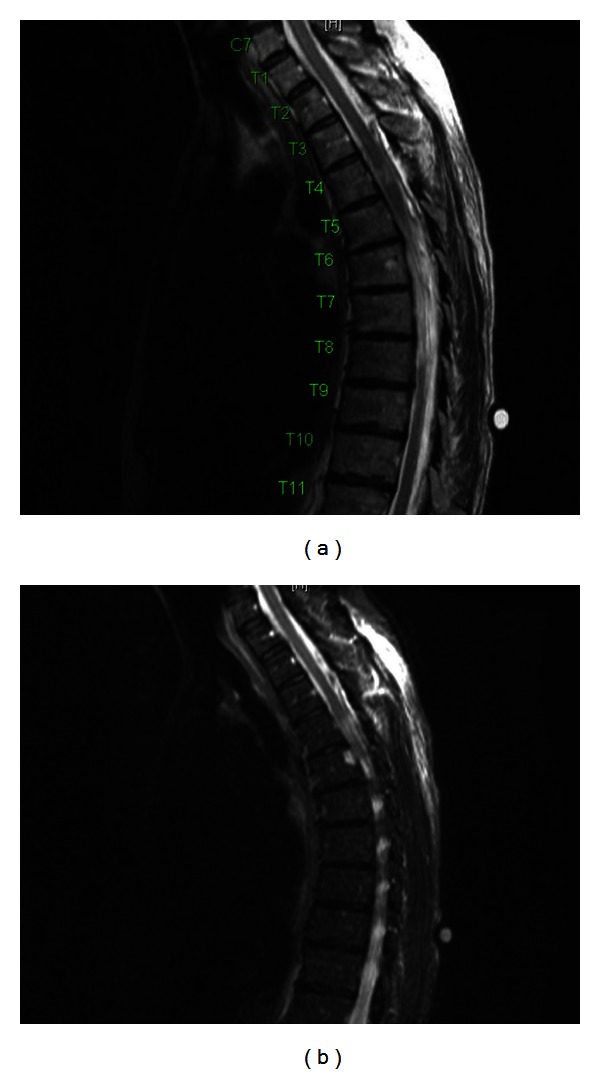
(a) Post-op sagittal T2 MRI with complete dural closure and complete tumor resection. (b) Post-op sagittal T2 MRI with complete dural closure and complete tumor resection.
